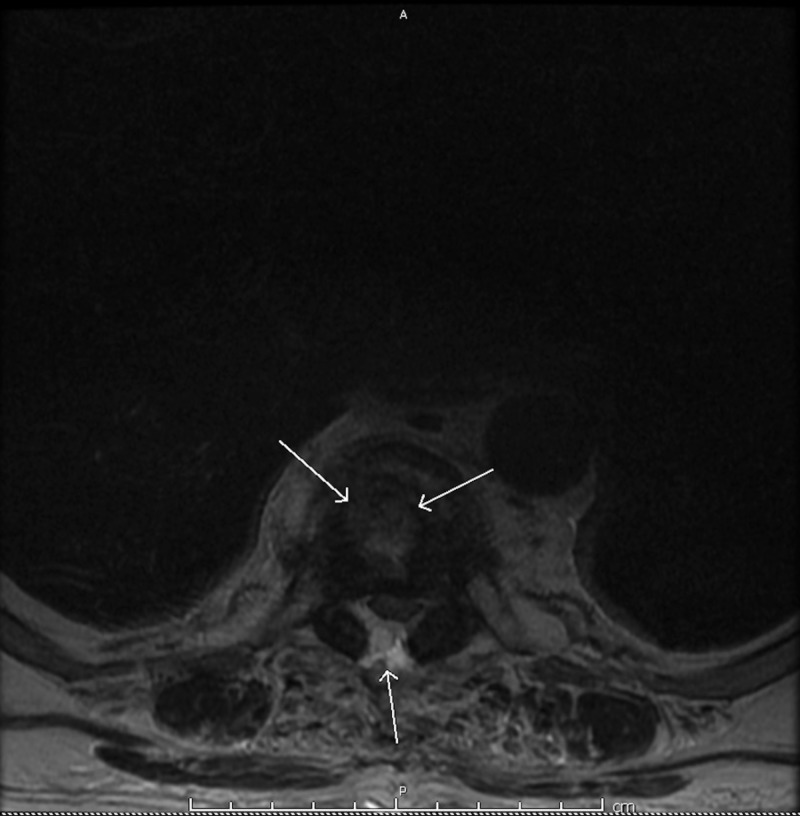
Transverse view of thoracic spine magnetic resonance imaging (MRI). MRI of thoracic spine with T7 discitis. Anterior arrows indicate inflammatory discitis while posterior arrow highlights spinal cord compression.
